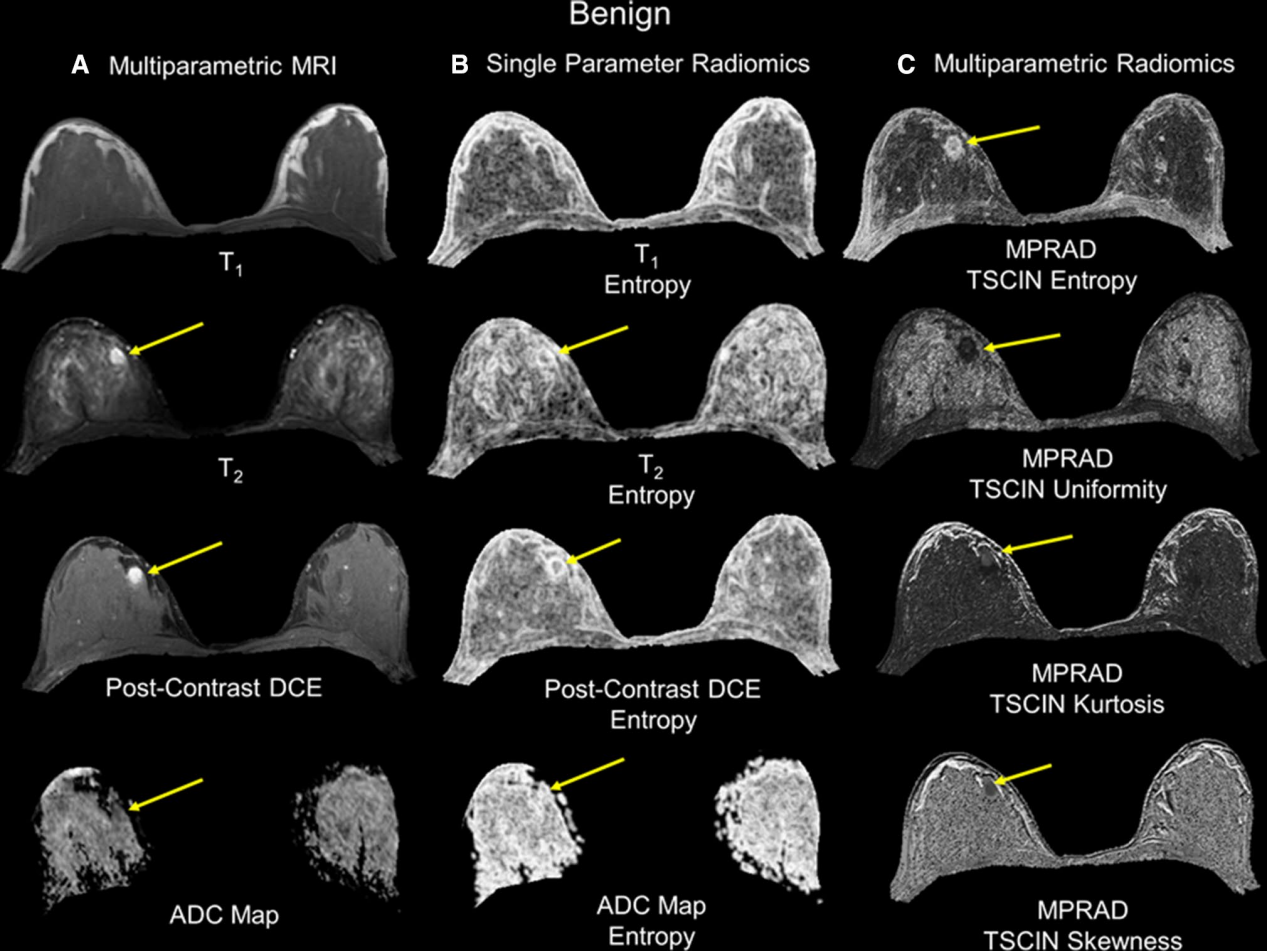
The radiomic feature maps (RFM) obtained from single and multiparametric radiomics (mpRad) analysis in a patient with a benign lesion. The straight yellow arrow highlights the lesion location. a Multiparametric MRI parameters used for the mpRad framework. b Single radiomic gray-level co-occurrence matrix (GLCM) entropy features maps from each MRI parameter. c The mpRad RFMs tissue signature co-occurrence matrix (TSCM) and tissue signature complex interaction network (TSCIN) radiomic features
